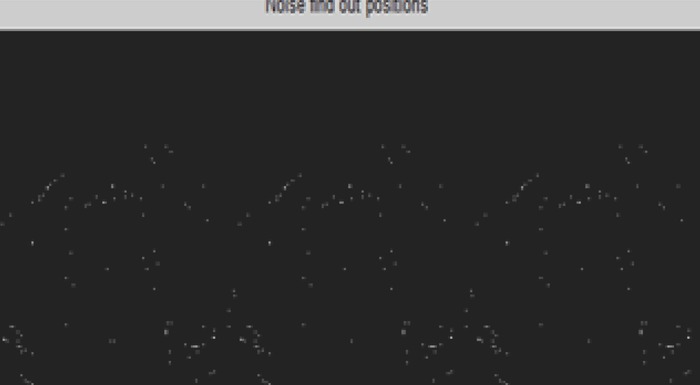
Noise find out position of 2-D CT image.
